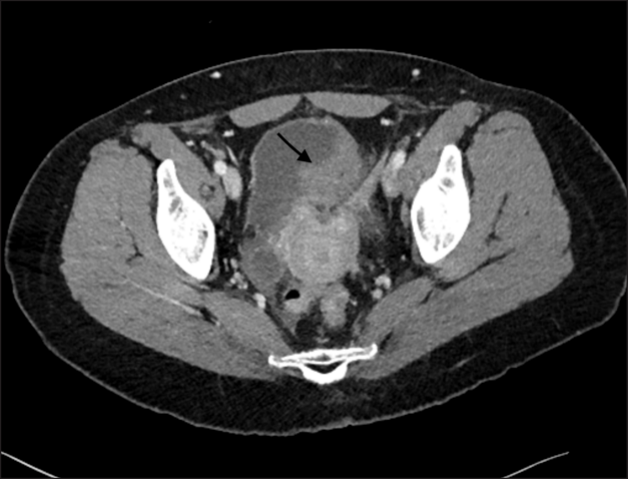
On a transverse CT image, the sentinel clot sign is quite subtle.
